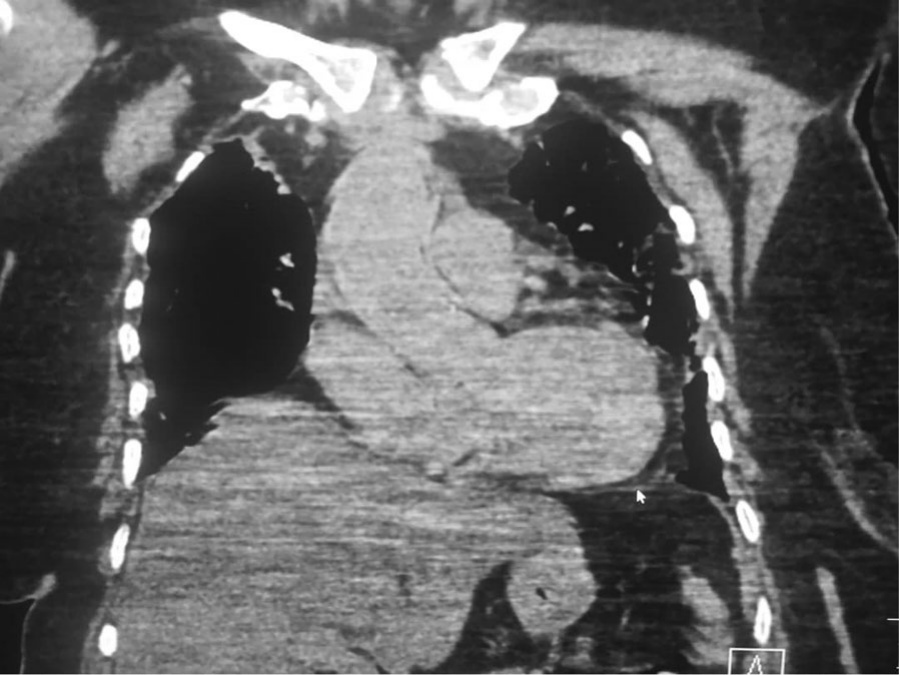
Coronal reformatted CT of the chest (mediastinal window) showing cardiomegaly with elevation of the cardiac apex (arrow), suggesting right ventricular dilatation
